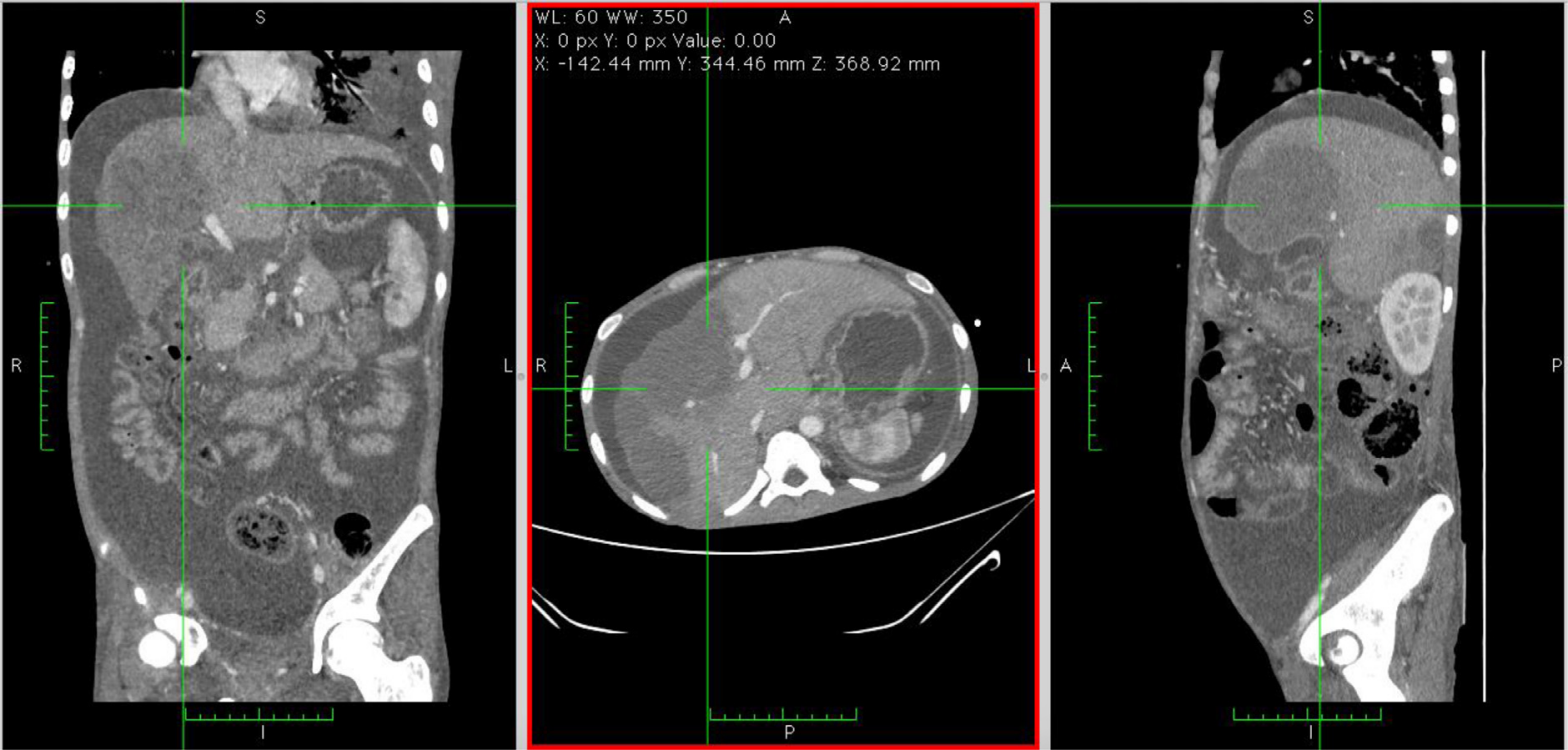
Abdominal CT scan at 3-month follow-ups after the treatment course; the liver underwent a noticeable recovery compared to the first examination.
